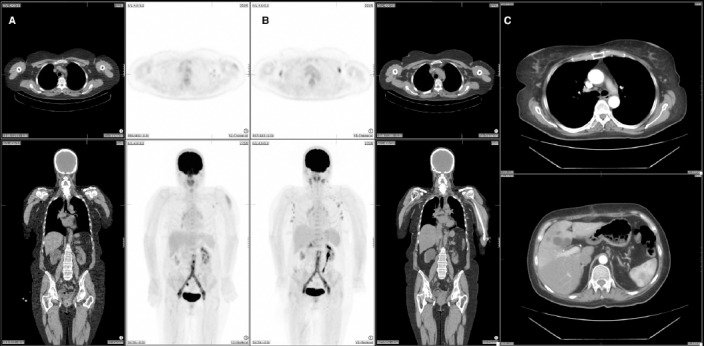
The first FDG-PET/CT study shows activity in the left deltoid and ipsilateral axillary lymph nodes (A). The second PET/CT study shows less but persistent FDG activity around the aortic graft as well as multiple FDG-avid lymph nodes on both sides of the diaphragm including bilateral cervical, bilateral axillae, porta hepatis, coeliac and left femoral regions (B). CT angiogram three months after removal of the infected graft, revealed no lymphadenopathy above or below diaphragm (C).
